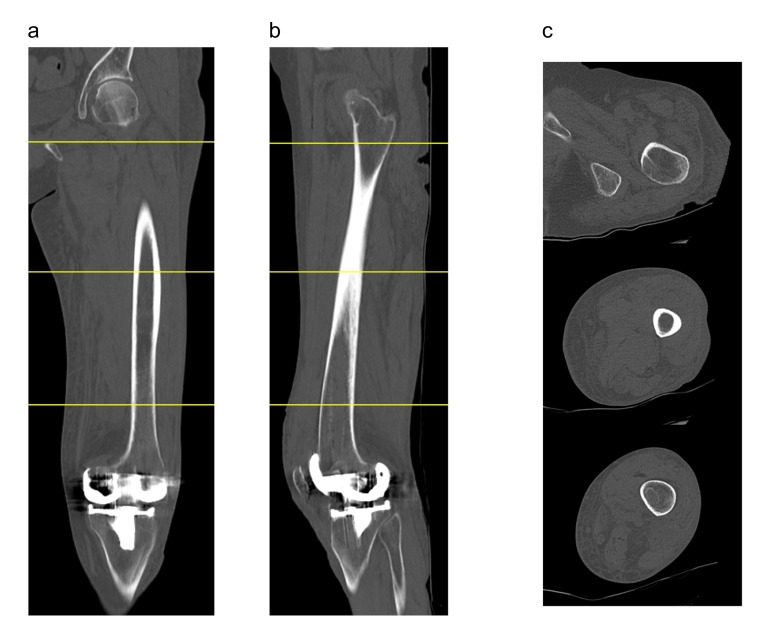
A CT scan was performed on the ninth day after the operation. a–c) The emphysema resolved.
